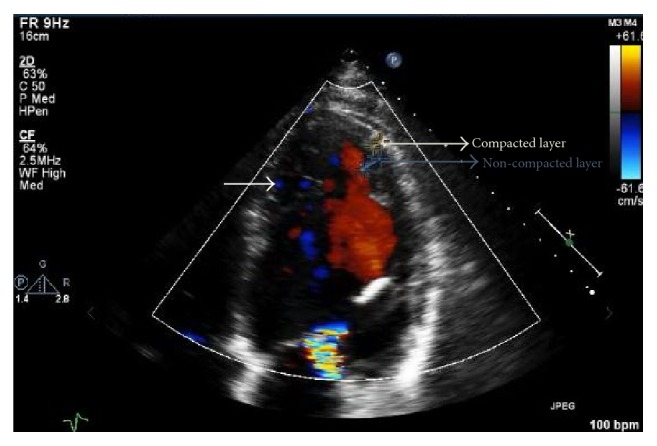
Color doppler echocardiogram demonstrating blood flow in the deep intertrabecular space (white arrow). Also, blue marking on the left ventricular wall showing noncompacted layer measuring 9.6 mm and yellow marking showing compacted layer measuring 4.4 mm with resulting ratio of noncompacted to compacted layer >2 at the end of systole.
